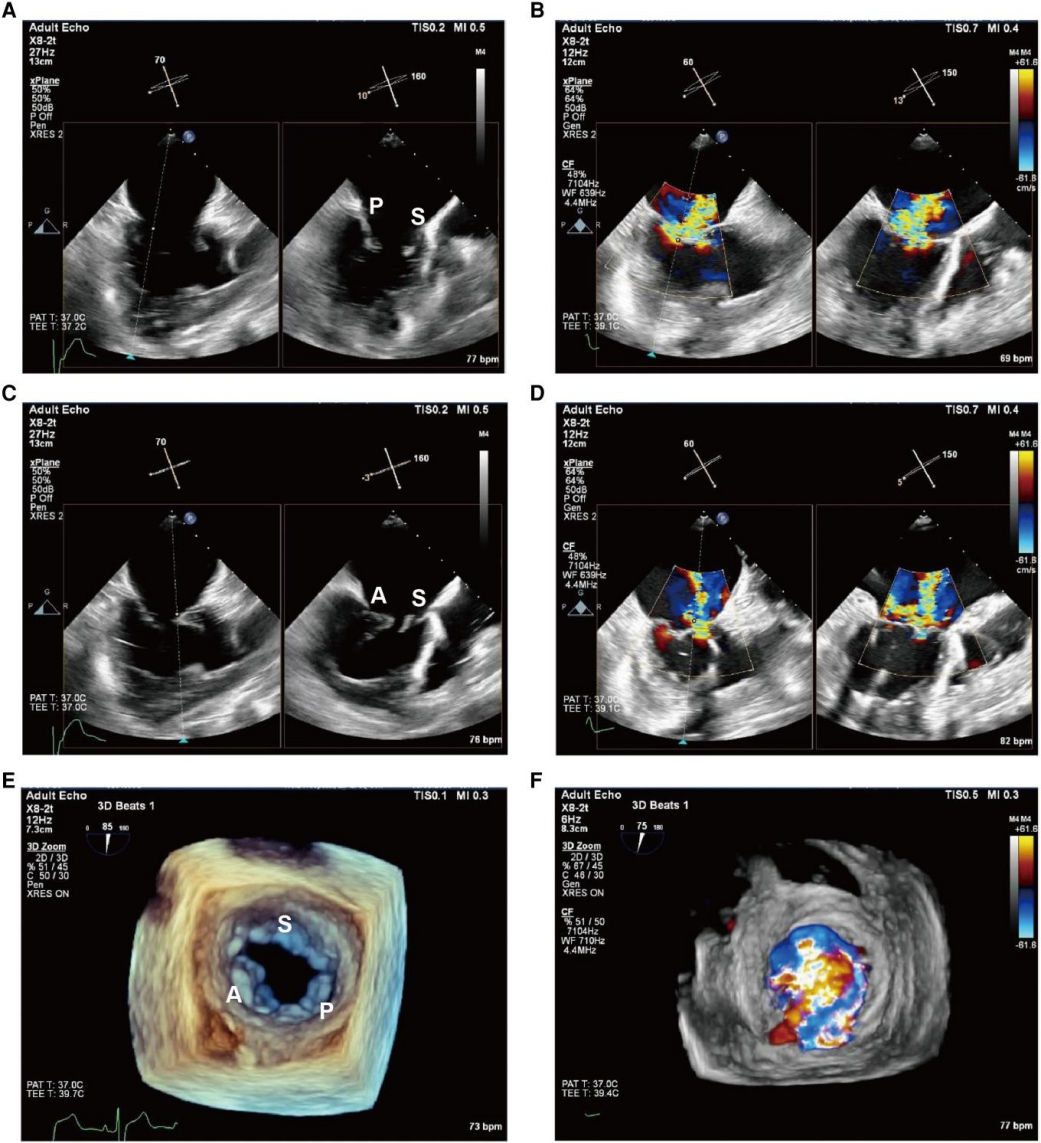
Transoesophageal echocardiography examination at mid-oesophageal. (*A–B*) Posterior–septal coaptation and significant regurgitation. (*C–D*) Anterior–septal coaptation and regurgitation. (*E–F*) Three-dimensional echocardiography demonstrating the leaflet anatomy, with regurgitation predominantly localized between the posterior and septal leaflets. A: anterior leaflet; P: posterior leaflet; S: septal leaflet.
